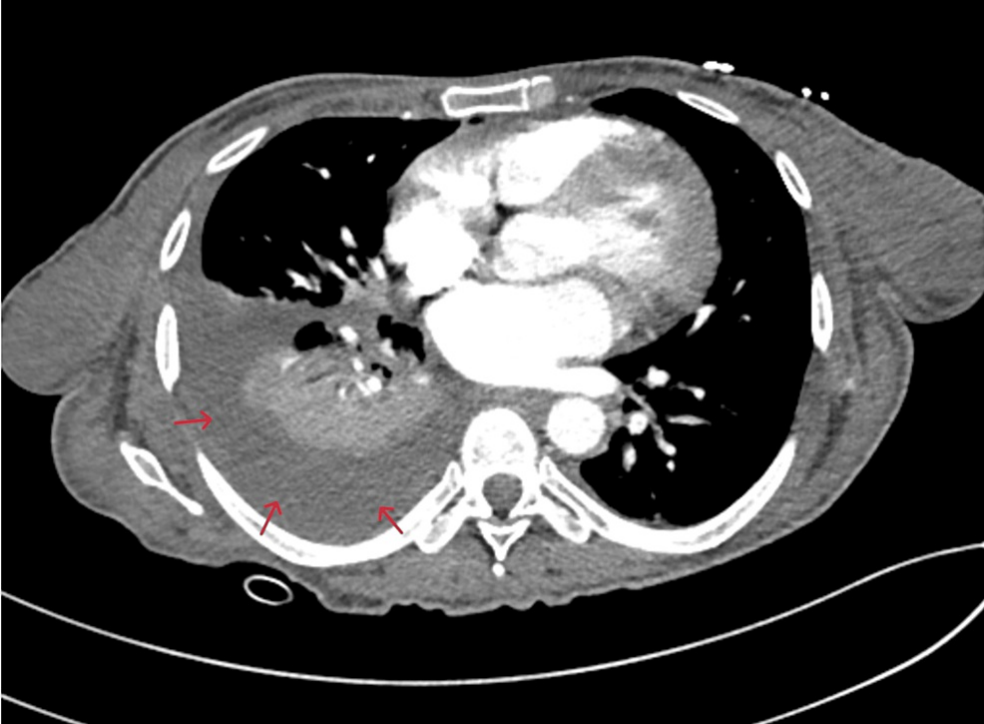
CT chest (axial view) shows dense consolidation in the right lower lobe and large right pleural effusion (red arrows).
